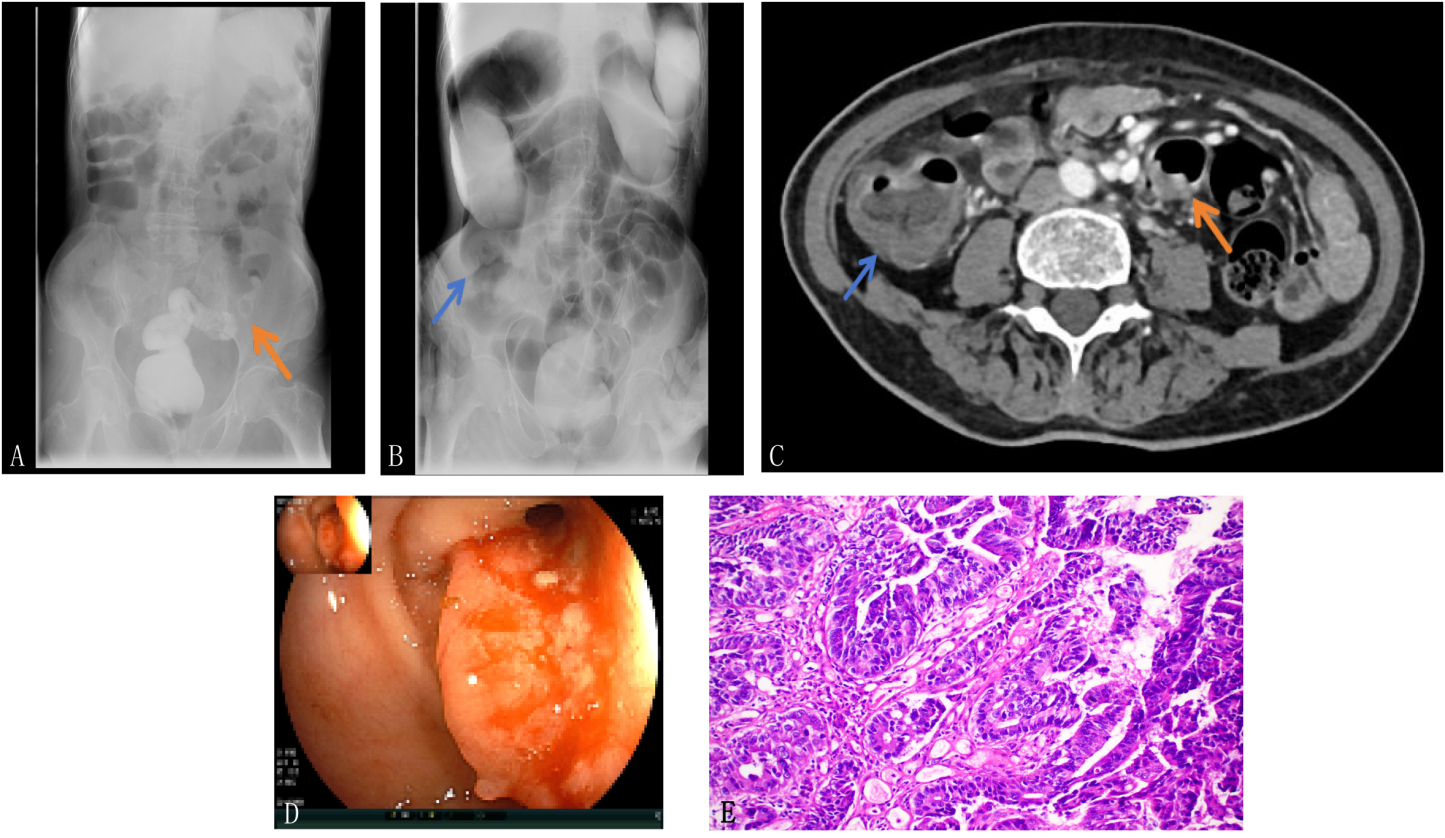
Preoperative examination. (A) Colonography revealed irregular filling defects in the sigmoid colon (→); (B) Colonography revealed irregular filling defects in the ileocecal region (→); (C) Abdominal CT scan with infiltrative mass and circumferential thickening in ascending colon and sigmoid colon (→); (D) Abdominal CT scan revealed an infiltrative mass with circumferential wall thickening in the ascending and sigmoid colon; (E) Biopsy examination revealed adenocarcinoma in the sigmoid colon lesion; Tissue slides: HE×20.
